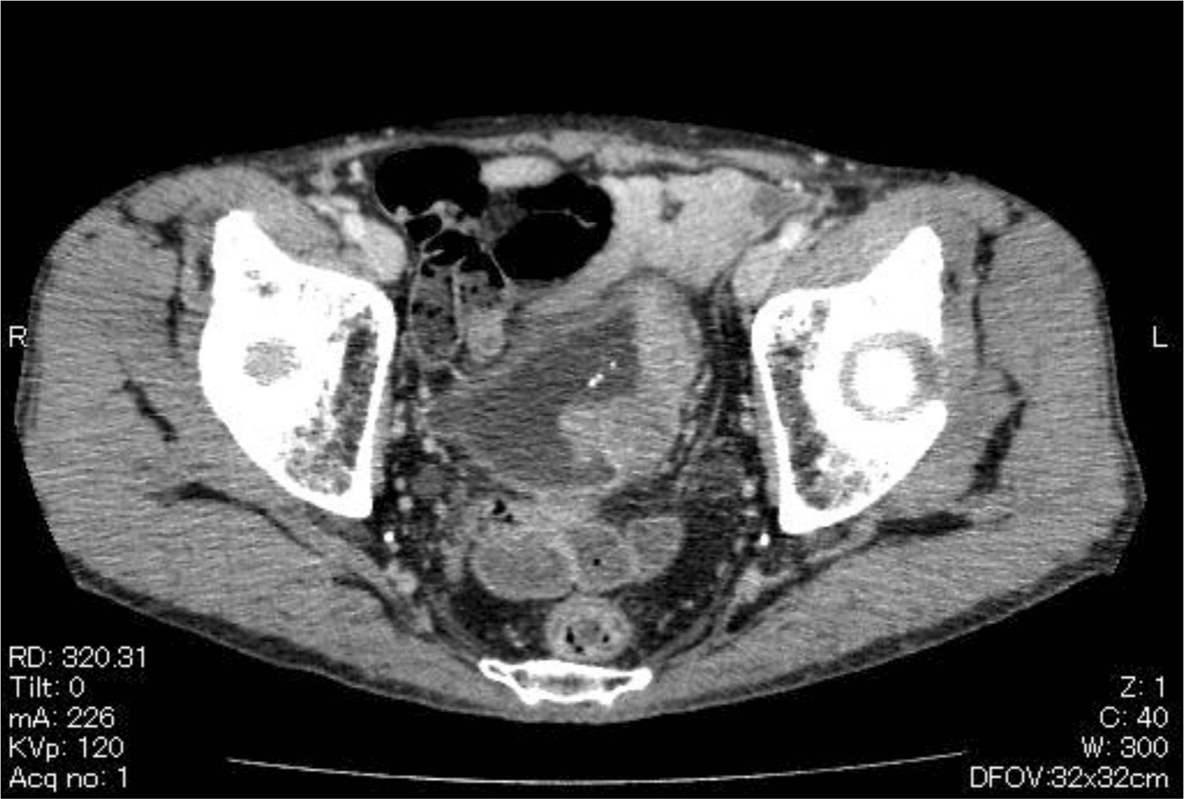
Preoperative CT findings of the pelvis; CT showed the invasive bladder cancer (clinical stage T3a) and the normal external iliac artery.
